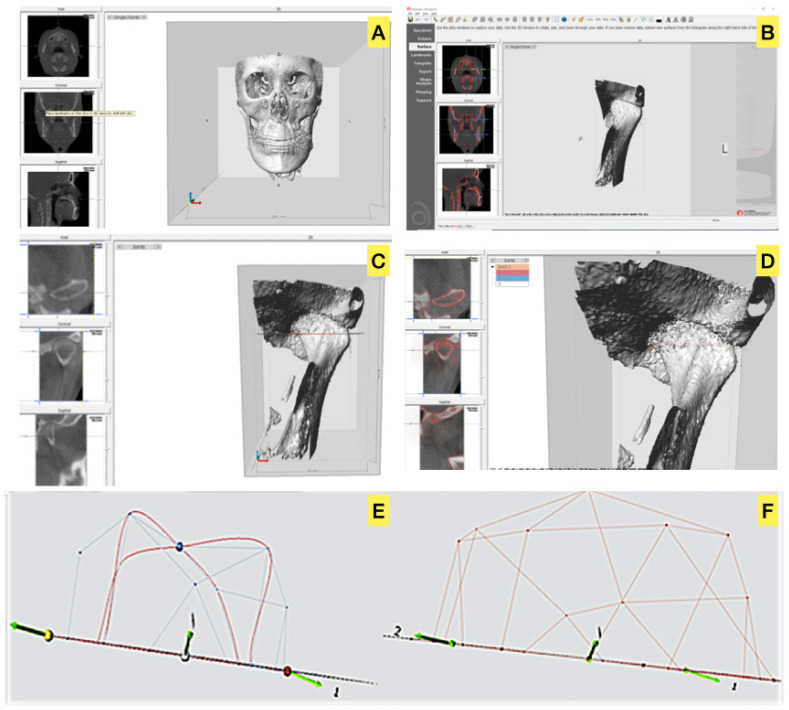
A view on Checkpoint (Stratovan): (A). Imported cone-beam computed tomography data exported in digital imaging and communications in medicine format, (B). Cropped image of the volume of the left condyle–fossa unit, (C). Individual points on the medial and lateral poles of the condylar head, (D). Distance measurement between the medial and lateral poles of the condylar head (diameter), (E). Volume space of the condylar head above the reference plane, (F). Volume space between the reference plane and glenoid fossa. We defined the difference between (E) and (F) as the joint space volume.
